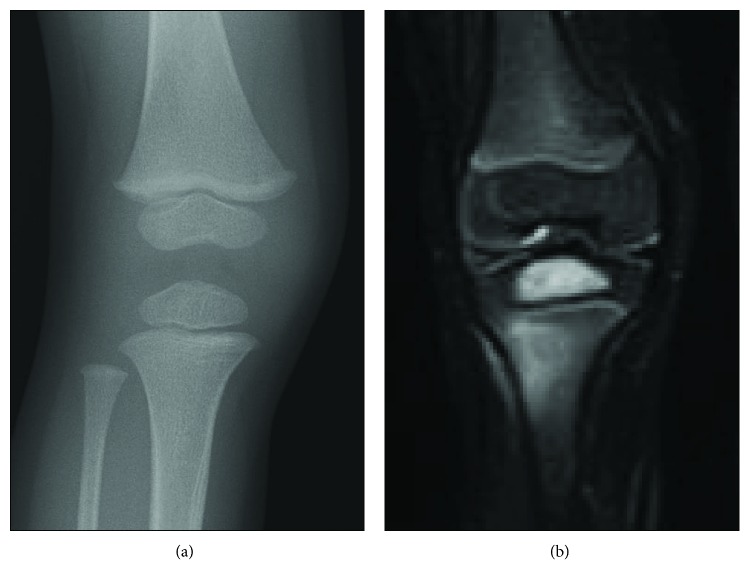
Initial examination. (a) Plain radiograph of the knee showing no apparent abnormalities. (b) MRI demonstrating a high-signal intensity area at the epiphysis of the right proximal tibia in the short tau inversion recovery sequence.
